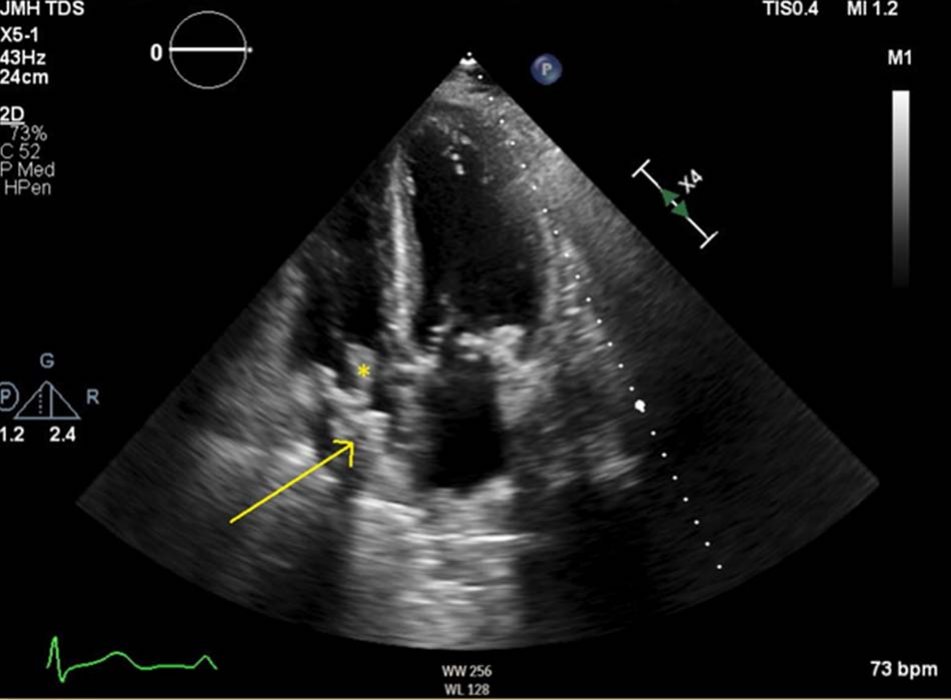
Apical four-chamber view showing thrombus in right atrium (arrow) with mobile element (asterisk).
